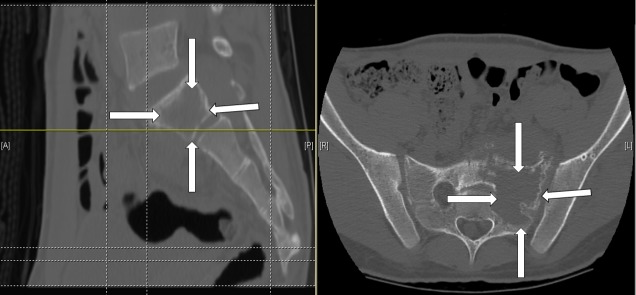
A preoperative CT scan showing the tumor mass invading the sacral bone (white arrows).
